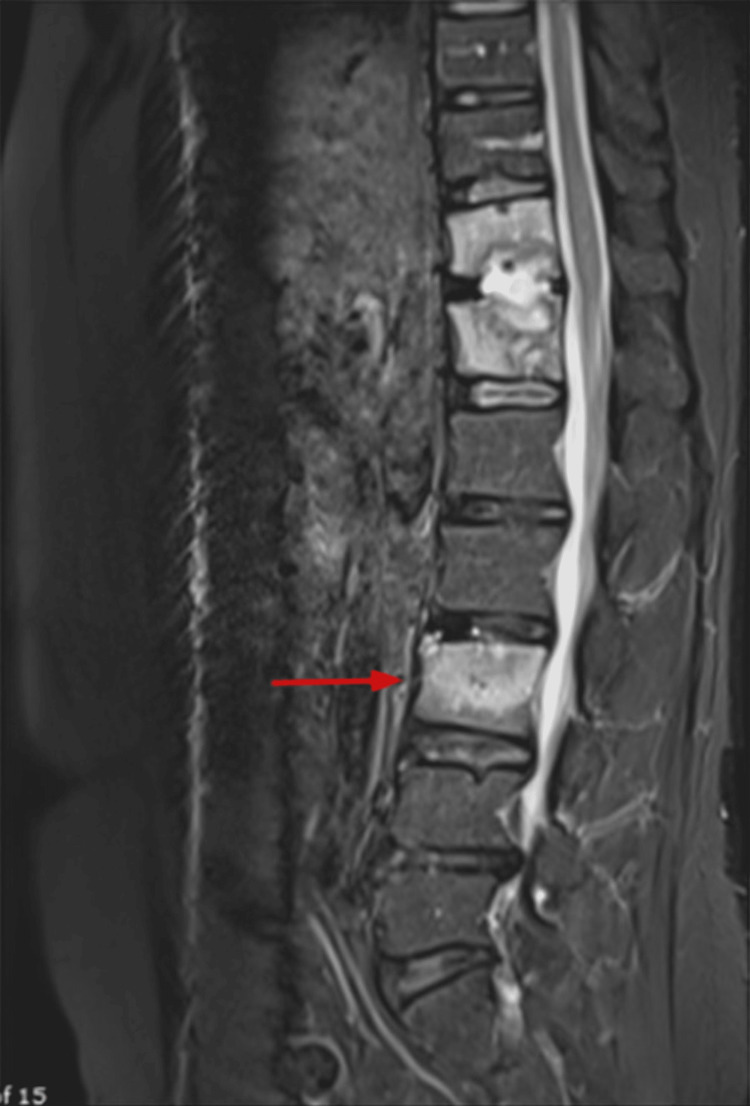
Sagittal MRI after two weeks of empiric antibiotic therapy Compared to Figures 2A and 2B, there is an increased bone marrow edema (red arrow) with no other significant changes.
